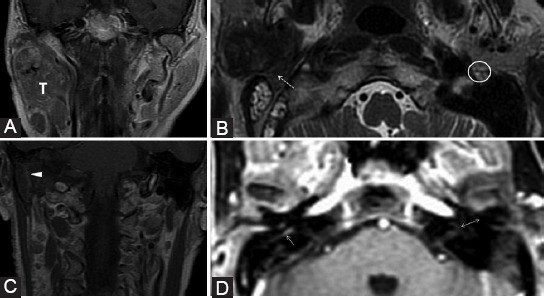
Parotid adenocarcinoma (T) of superficial and profound lobes, extending in the area of facial nerve distribution; the patient presented with the right peripheral facial palsy. Axial T2-weighted image (B) shows right stylomastoid foramen fat effacement (dashed arrow) versus the left side (ellipse). Coronal contrast-enhanced T1-weighted images (A, C) reveal retrograde PNS along the mastoid segment of the right CN VII that shows CE (arrowhead). Dynamic contrast enhancement sequence (D) demonstrates possible upper involvement of the CN VII as there appears asymmetric CE of the right tympanic segment (arrow) when compared to the left side (double arrow).
